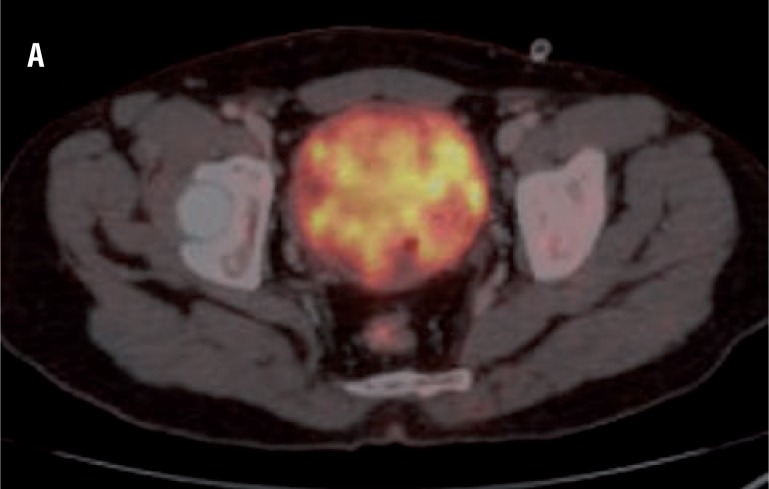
High FDG uptake is seen in soft tissue mass in the bladder suggestive of primary lesion.
